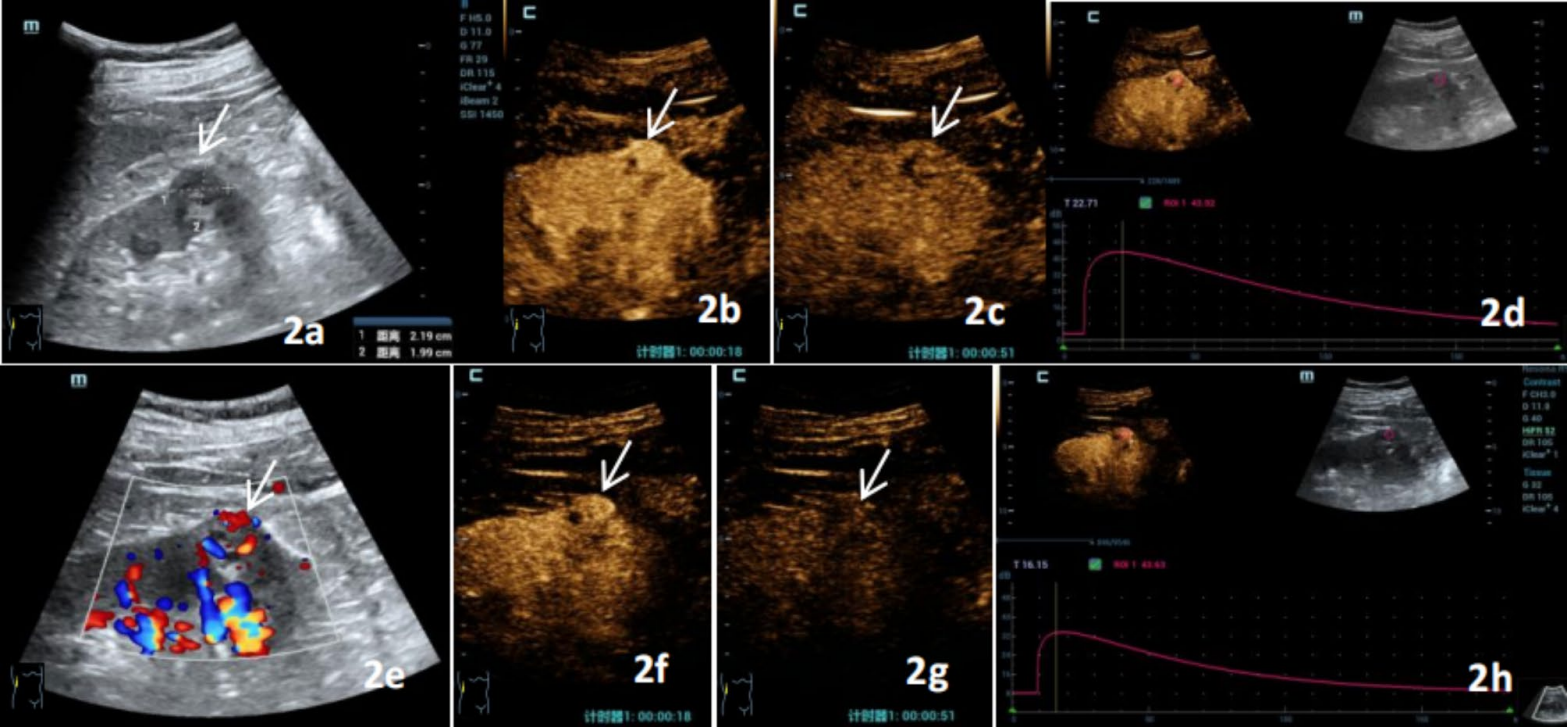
A 45-year-old male patient with clear cell renal cell carcinoma, gray-scale ultrasound, C-CEUS, and H-CEUS images. 2a: Conventional ultrasound shows extremely hypoechoic mass under the right kidney, with a size of 2.19 cm × 1.99 cm, a clear boundary, and a regular shape. 2b and d show C-CEUS images. 2b: Diffuse enhancement of both periphery and center of the lesion at 18s during perfusion period. 2c: Hermogeneous high enhancement at 51 s in the wash-out period. It shows 'fast advance and slow retreat’. 2d: C-CEUS time-intensity curve. 2e: CDFI shows grade III blood flow signal in the lesion. 2f and h show H-CEUS images. 2f: Hermogeneous high enhancement at 18s during perfusion period. 2g: Hermogeneous low enhancement at 51s during wash-out period.It shows ‘fast advance and fast retreat’. 2h: H-CEUS time-intensity curve (arrow shows lesions)
